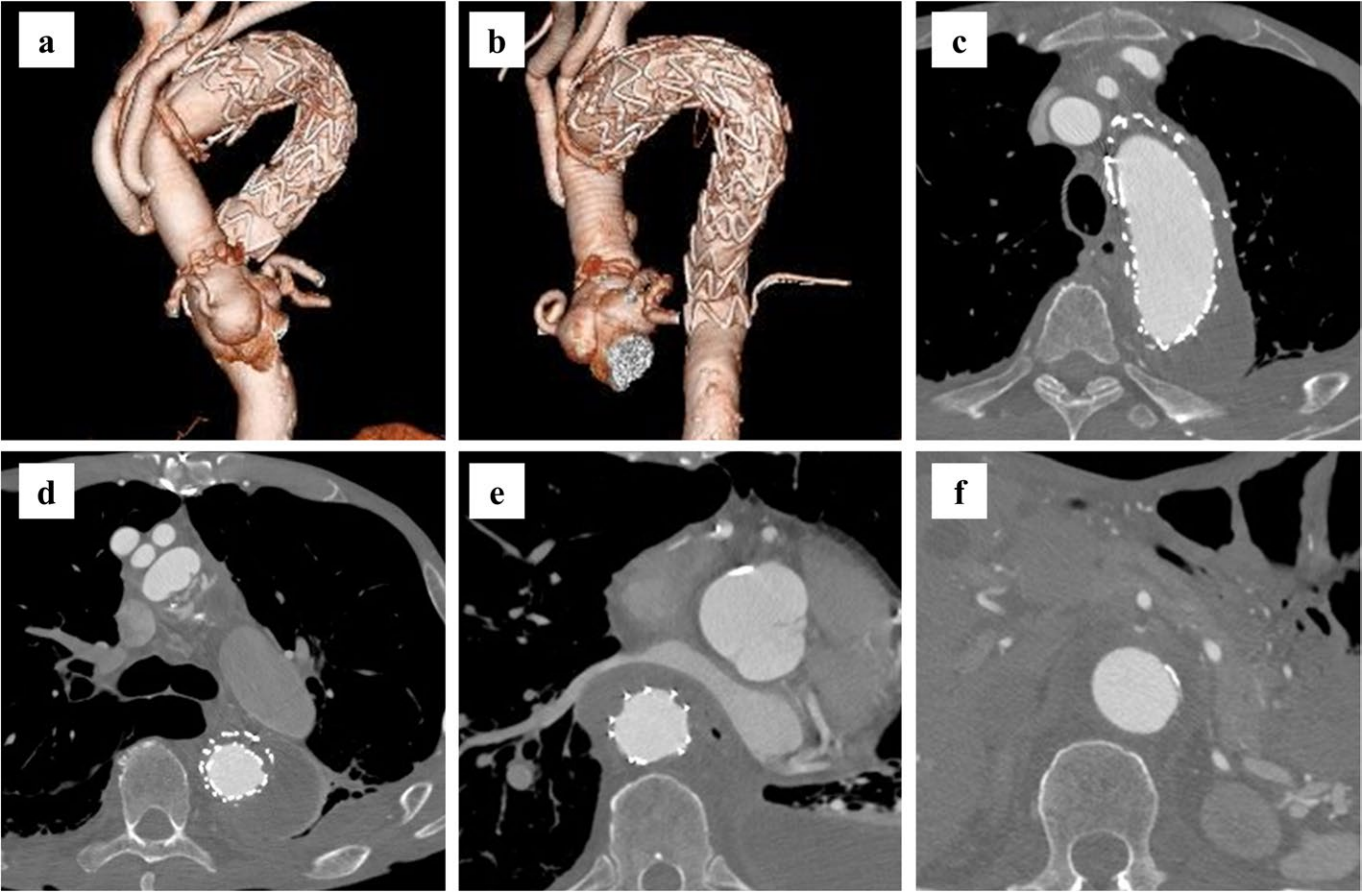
Contrast-enhanced computed tomography at two months after surgery. a, b Three-dimensional imaging of ascending to descending aorta. c, d The false lumen is completely thrombosed and shrunk. e The compressed stent graft improves. f The re-entry above the celiac artery has disappeared, and the false lumen is shrunk
